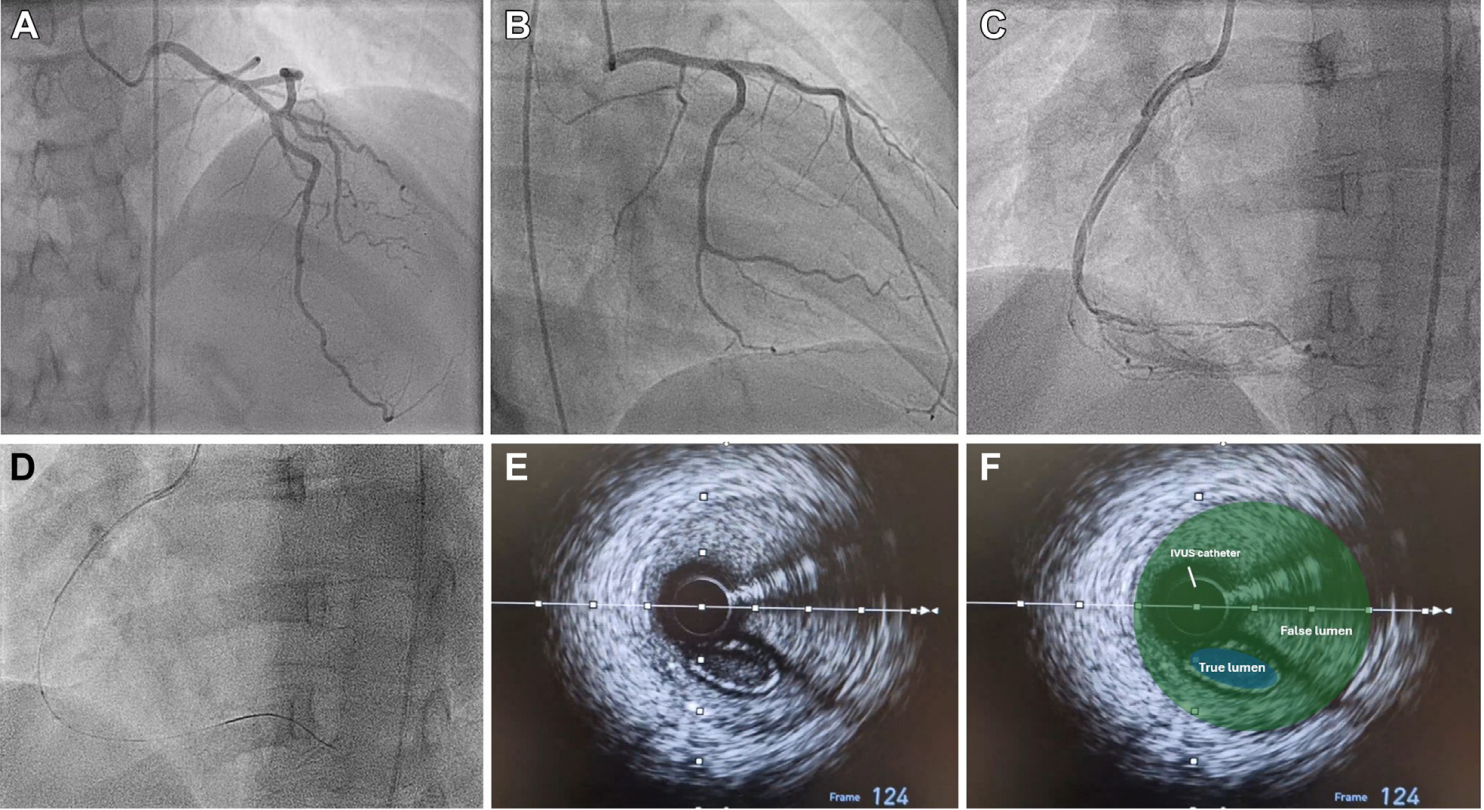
Coronary Angiography and Intravascular Ultrasound Coronary angiography demonstrating no significant disease in the (A) left anterior descending artery and (B) left circumflex artery. (C) On engagement of the right coronary artery, the catheter caused a spiral dissection. (D) Coronary wire passed into the right coronary artery with intravascular ultrasound (IVUS) performed over the coronary wire. (E and F) Intravascular ultrasound shows the wire and intravascular ultrasound catheter with a large false lumen.
